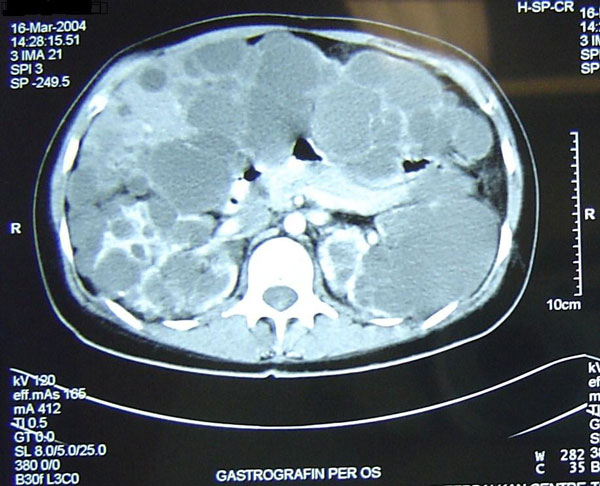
Pre-operative computed tomography demonstrating multiple cysts in the liver, anterior surface of the pancreas and kidneys.
